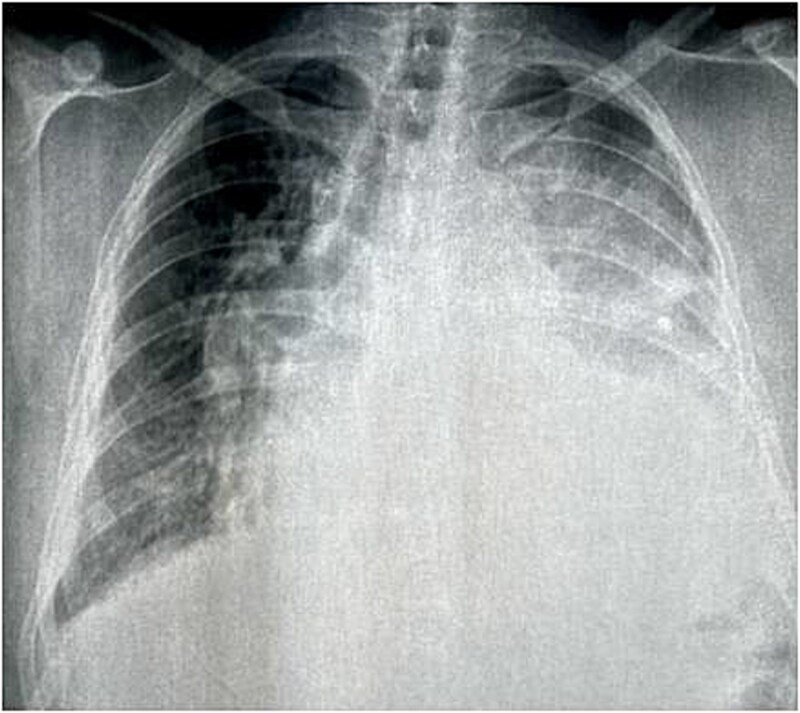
Chest X-ray showed a left opacity that encompassed the cardiac outline and displaced the trachea and stem bronchi on the right.
